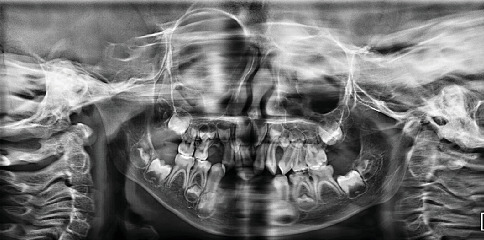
Panoramic radiograph at 4 years 11 months. The image demonstrates dentition with multiple deep dentinal caries. Atraumatic restorative treatment was performed on affected teeth. Due to the patient's limited cooperation, the radiograph quality is suboptimal, yet diagnostic information could still be obtained. Possible germ absence of Teeth LL5 and LR5 was suspected at this stage.
